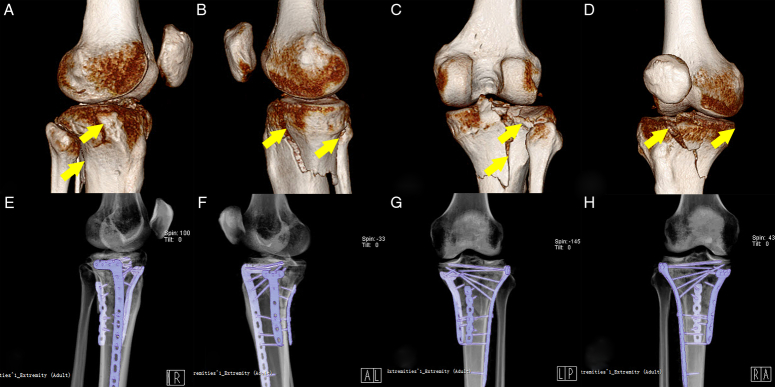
The therapeutic protocol for multiquadrant fractures. (A–D) The preoperative 3-D CT images demonstrate fractures in all four quadrants. A: AL+PL fractures. B: AM+PM fractures. C: PM+PL fractures. D: AM+PM fractures. (E–H) Postoperative volume reconstruction images show that the anatomical reduction of all four quadrants was achieved using the ‘three-column’ fixation concept. In this case, it involved using a large lateral buttress-rafting plate for overall stability, a PM buttress plate for the buttressing effect, and a PM reconstruction plate for the antiglide effect. AL, anterolateral; AM, anteromedial; PL, posterolateral; PM, posteromedial.
